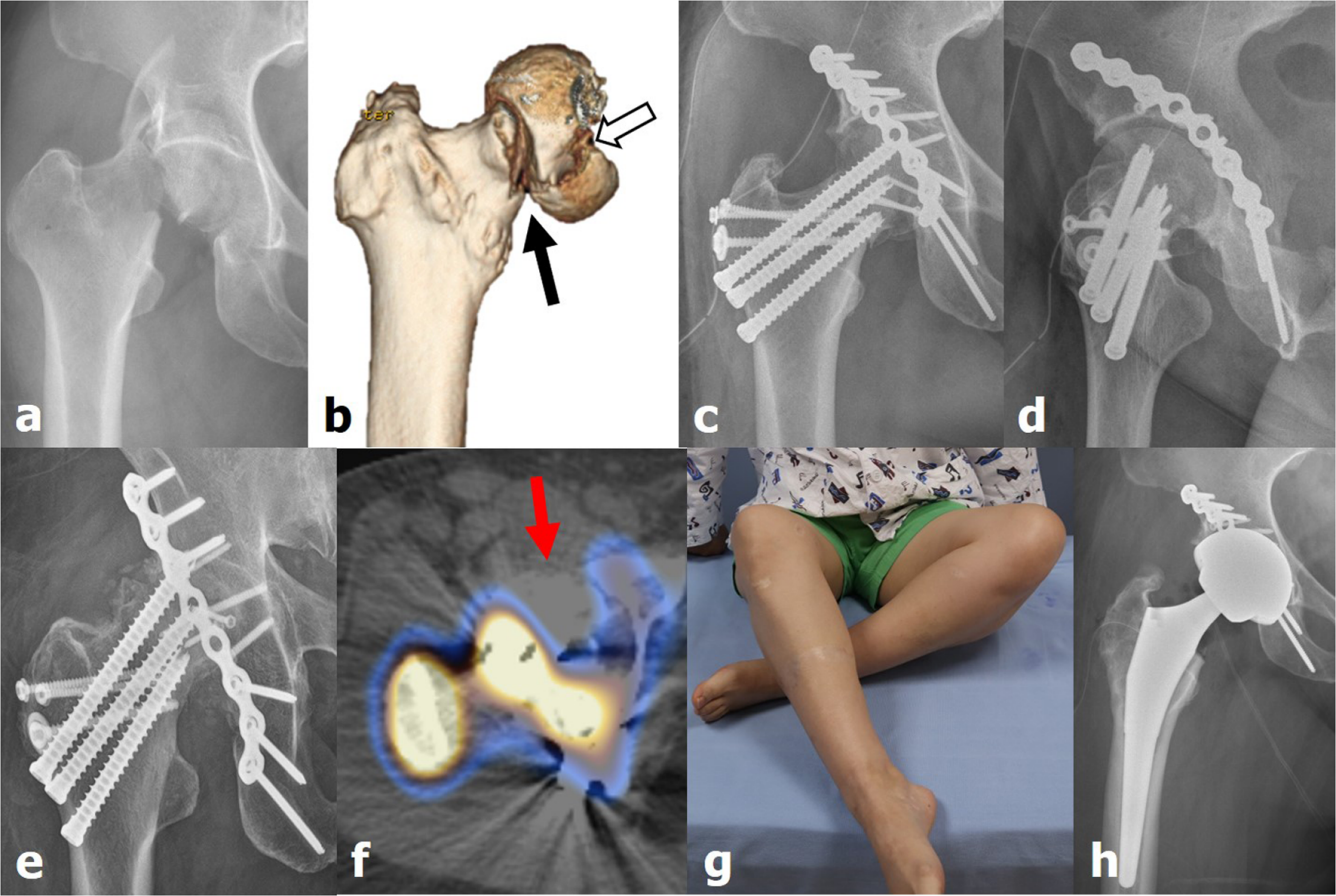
(a, b) Pipkin type IV femoral head fracture and dislocation associated (white arrow) with a displaced femoral neck fracture (black arrow) of the right hip joint owing to an in-car traffic accident (case 34, a 47-year-old woman). (c, d) After performing surgical hip dislocation with trochanteric flip osteotomy, the femoral neck and head were fixed and compression plating was performed for the acetabular fracture. (e, f) Bone union was achieved 8 months after surgery; however, reduced blood flow (red arrow) in the femoral head was observed on single-photon emission computed tomography/computed tomography. (g, h) The patient complained of limited range of motion and pain in the right hip joint, and eventually underwent total hip arthroplasty
